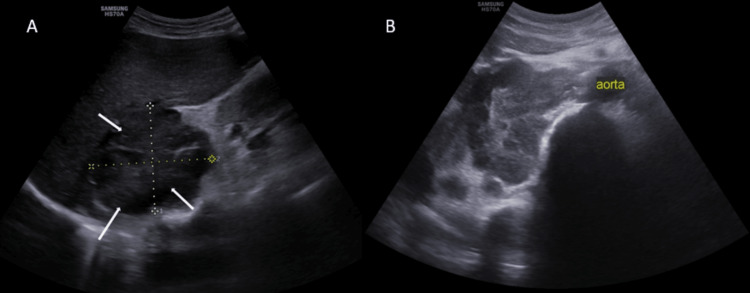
A: Abdominal ultrasound showing right adrenal mass. B: Abdominal ultrasound showing right para-aortic lymph nodal mass.
